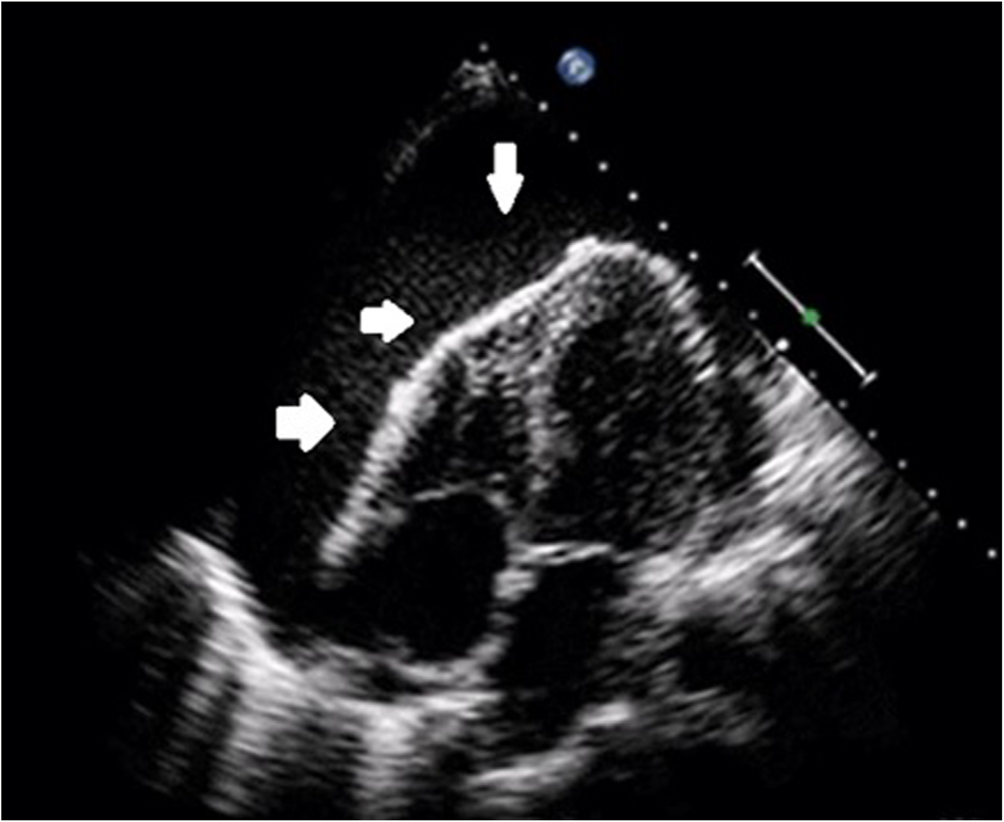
Four-chamber echocardiography still demonstrating large global pericardial effusion (white arrows).
